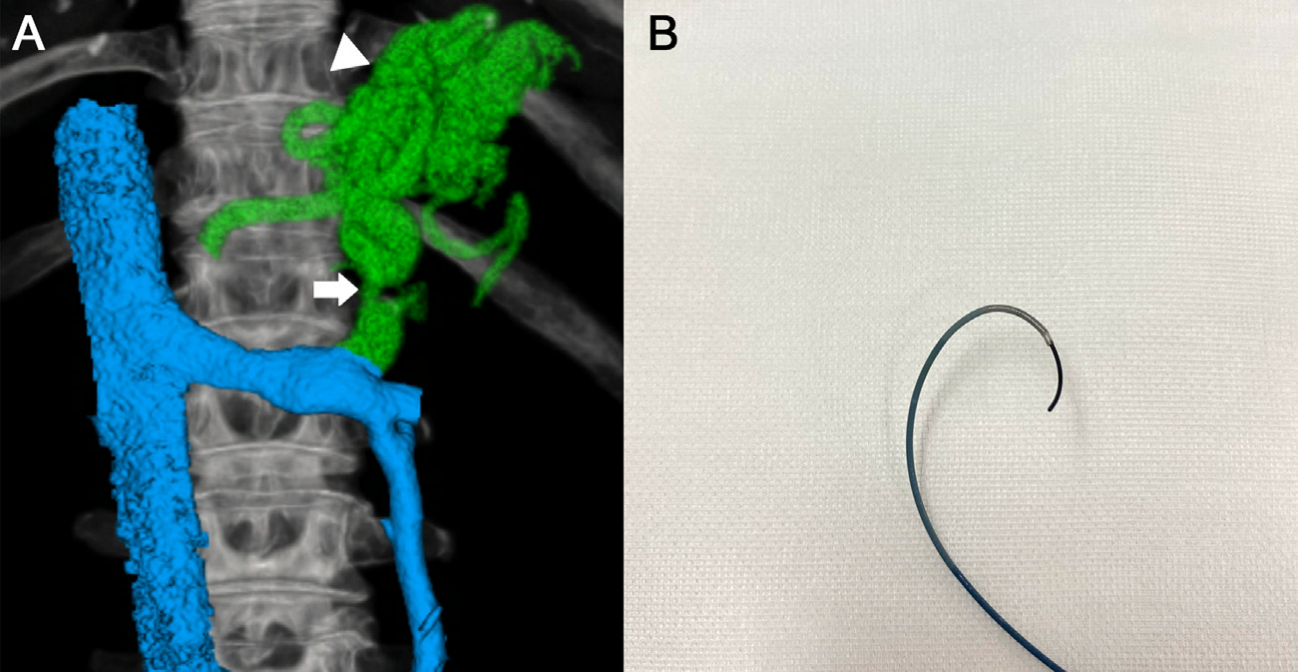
3D VR image reconstructed from CE-CT before BRTO, and steerable-wire. (A) A GR shunt (arrow) and gastric varices (arrowhead) were observed. (B) Both a high-flow steerable catheter and a 0.025-inch guidewire were used as a steerable-wire. 3D VR, 3-dimensional volume rendered; CE-CT, contrast-enhanced computed tomography; BRTO, balloon-occluded retrograde transvenous obliteration; steerable-wire, a high-flow steerable microcatheter and a 0.025-inch guidewire combination; GR shunt, gastro-renal shunt.
